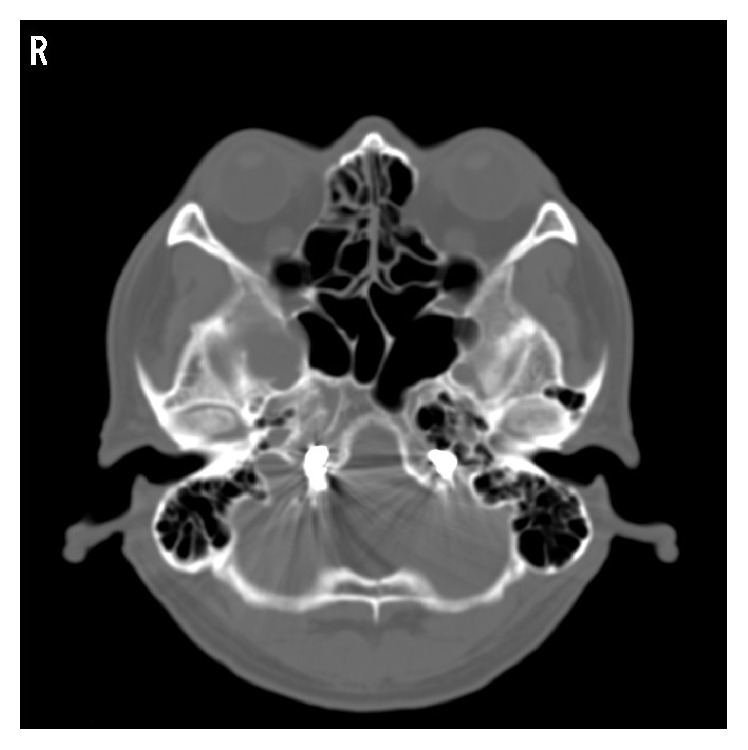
CT after treatment demonstrates packing coil seated in right ACC to hypoglossal canal (treated 5 years ago) and in left ACC.
